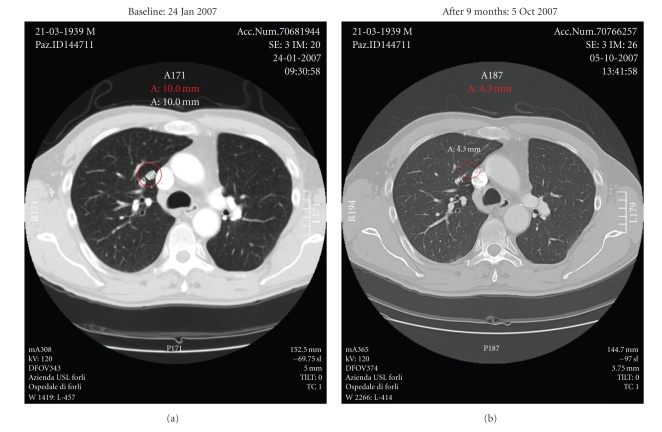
CT scan of patient *n. *25 (BI) before and at the end of vaccination cycles. The red circle indicates the PR observed to vaccine. The patient underwent palliative surgery following progression in adrenal gland and abdominal lymph node metastases after stopping vaccination and has ongoing PR in the lung.
